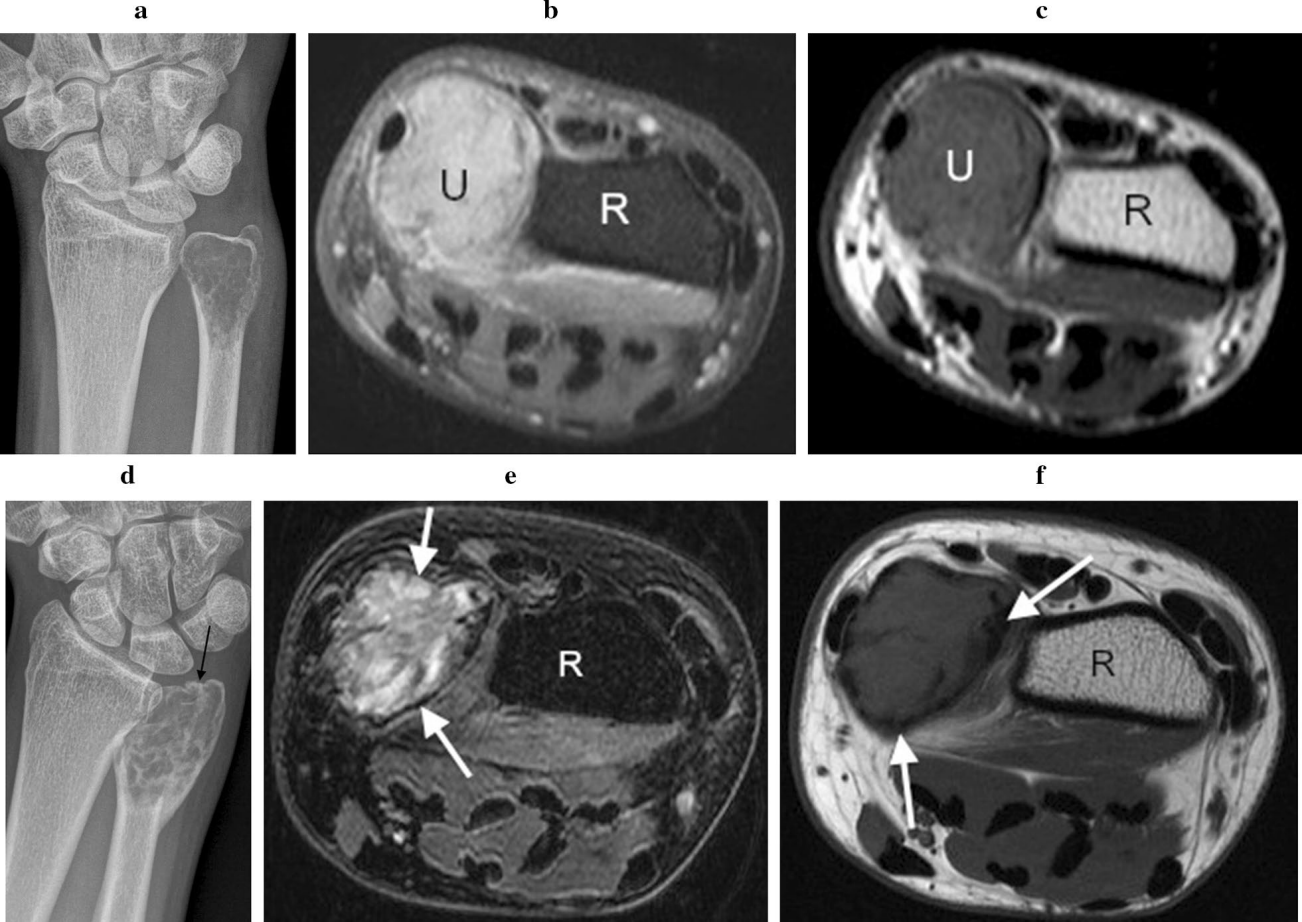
Patient 3: pre denosumab, a frontal plain radiograph demonstrates a Campanacci grade 2 distal ulnar GCTB with mild expansion and cortical thinning. b Axial T2-weighted fat saturated and c axial T1-weighted MR images confirm an expansile heterogeneous predominantly high T2- and low to isointense T1-weighted distal ulnar lesion (*U* ulnar, *R* radius). Post denosumab at 2 months, d frontal plain radiograph shows marginal sclerosis and internal matrix sclerosis, with mild modeling deformity of the ulnar articular surface (black arrow). e Axial T2-weighted fat saturated and f axial T1-weighted MR images show definition of cortical margins with the formation of marginal sclerosis seen as peripheral low T1 and T2-W signal (white arrows). The tumour matrix is more heterogeneous with areas of low T2-W signal reflecting internal matrix consolidation (*R* radius)
